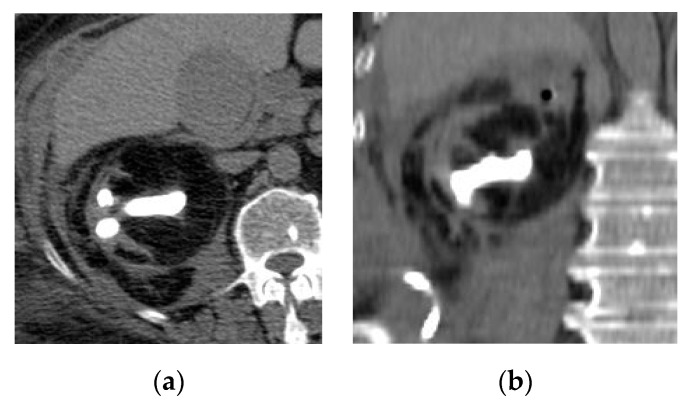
Right renal sinus lipomatosis. (a) Axial and (b) coronal reformatted non-contrast CT images show small chronic pyelonephritic right kidney with branched hyperdense stone at the renal pelvis and middle calyx. There is a large fatty area of low density replacing the renal sinus and involving the renal parenchyma and the perirenal space.
